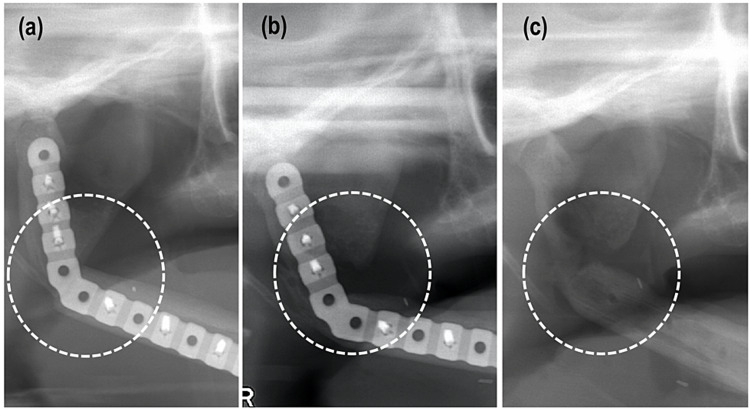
Recurrence at the resection margin in patient 12. (a) Panoramic radiographic images 1 month after surgery. (b) Bone resorption was evident 2 years after surgery. (c) After plate removal, bone union was confirmed.
